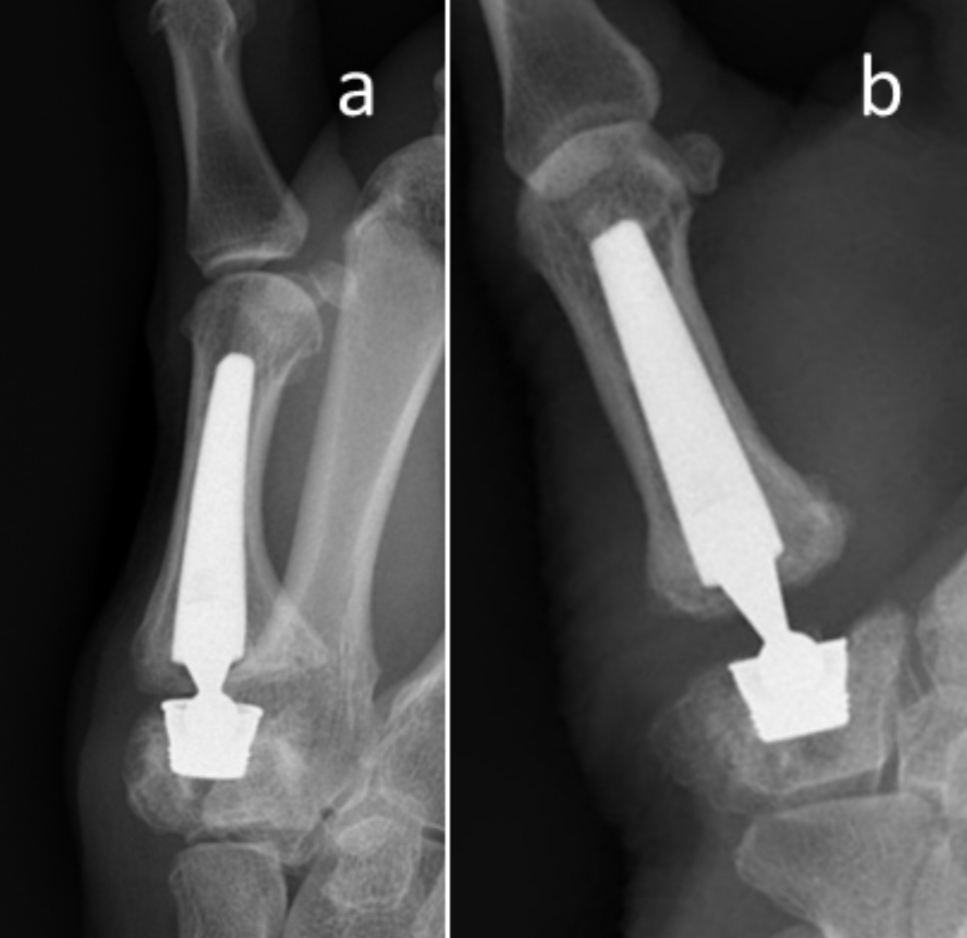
Radiographic imaging of adverse events in 1 patient with a MOOVIS prosthesis before and after revision surgery. Patient 1: (a) Restricted movement, treated with exchange of the neck component to a larger size (b) 15 months after primary surgery
